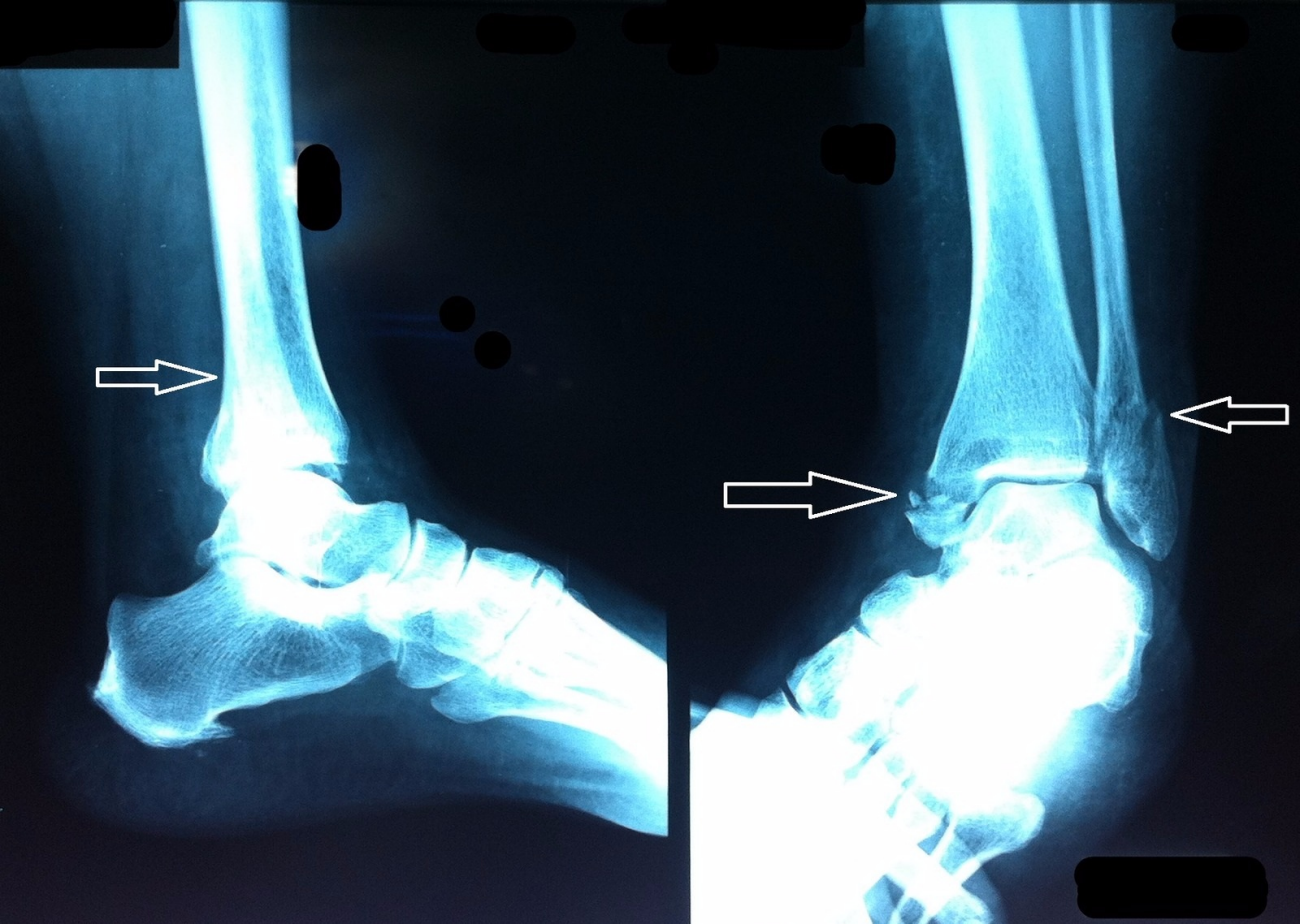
X-ray left foot Left trimalleolar fracture involving the lateral malleolus, the medial malleolus, and the distal posterior aspect of the tibia (white arrows).
